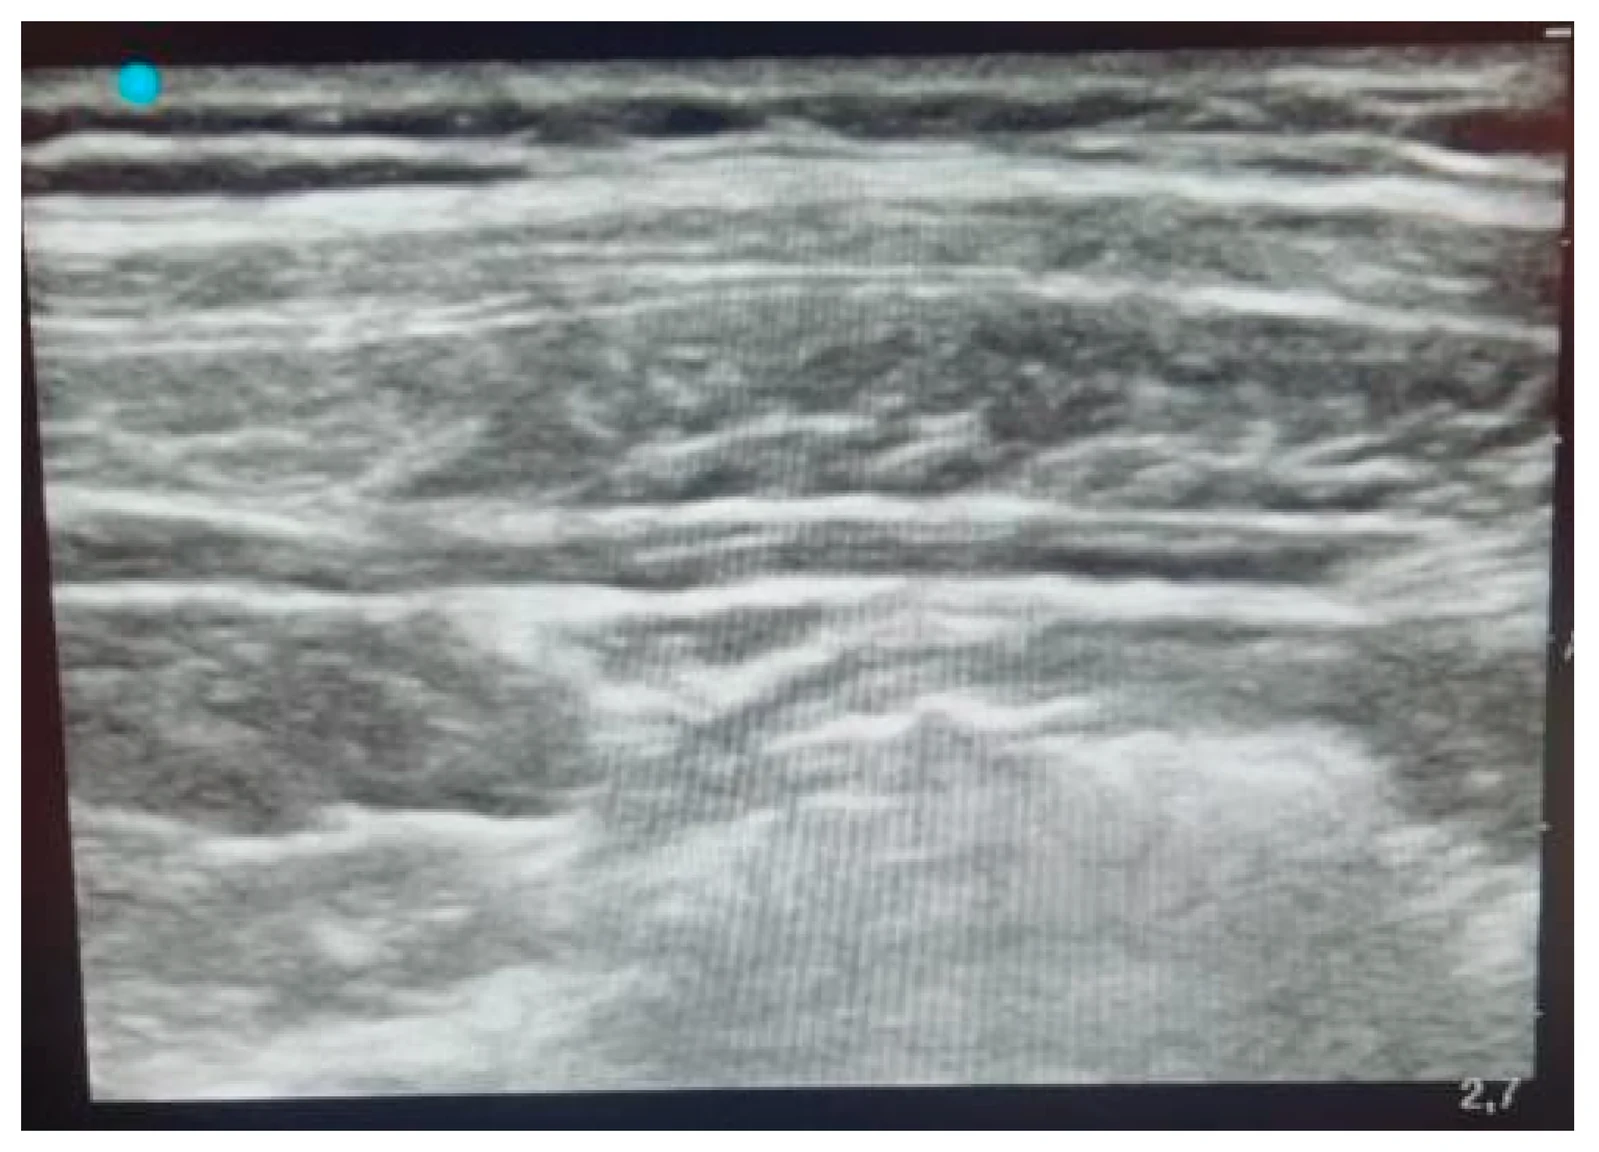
Ultrasound-guided creation of retroperitoneal space.
